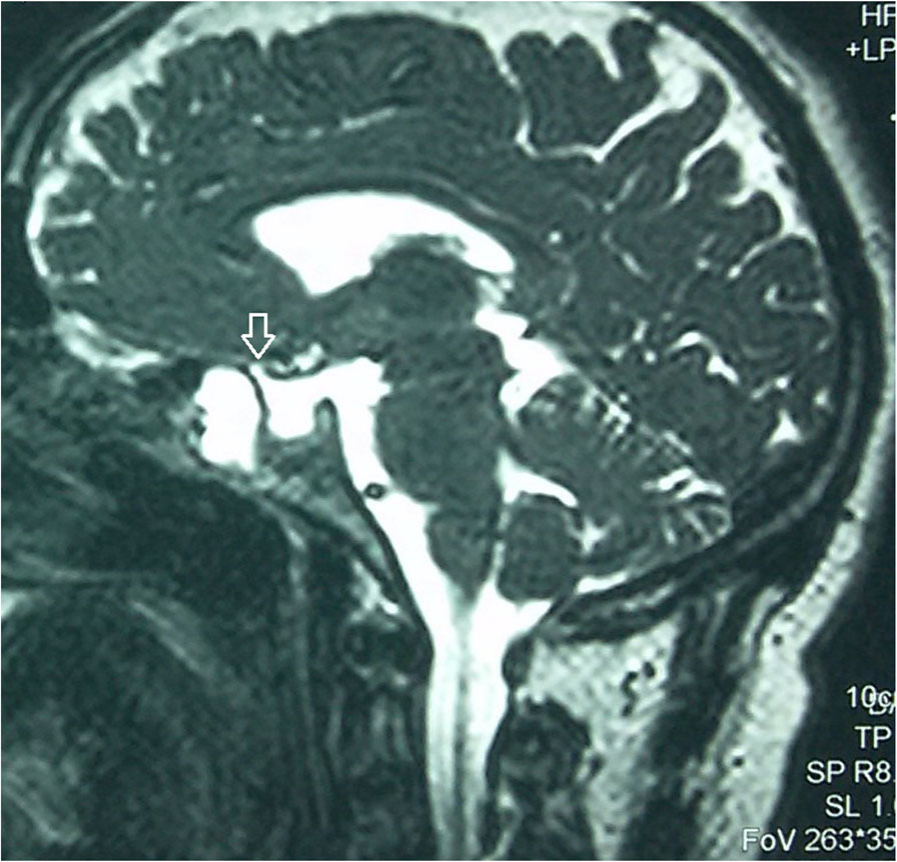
T2-weighted magnetic resonance imaging scan (sagittal cut) showing an empty sella associated with a pituitary arachnoidocele and fistula (*arrow*)
